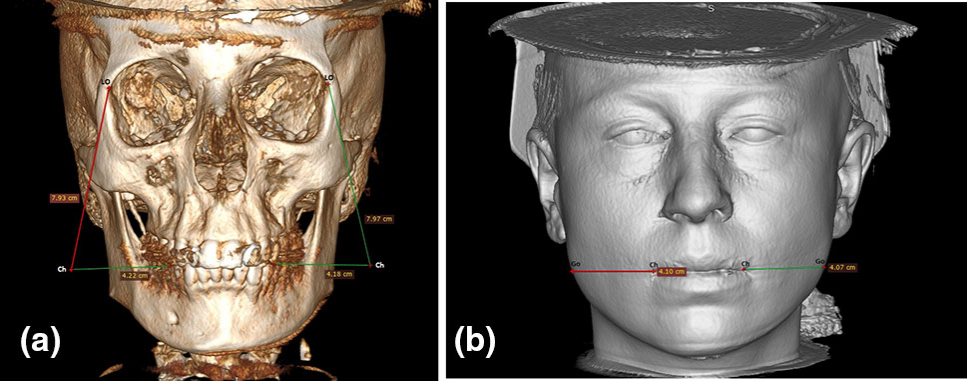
The measurement of distances between Ch-Go and LO-Ch on both sides of the face were depicted through 3D CT skin format (a) and 3D CT bone format (b). Ch: Chellion, Go: Gonion, LO: Lateral orbit
